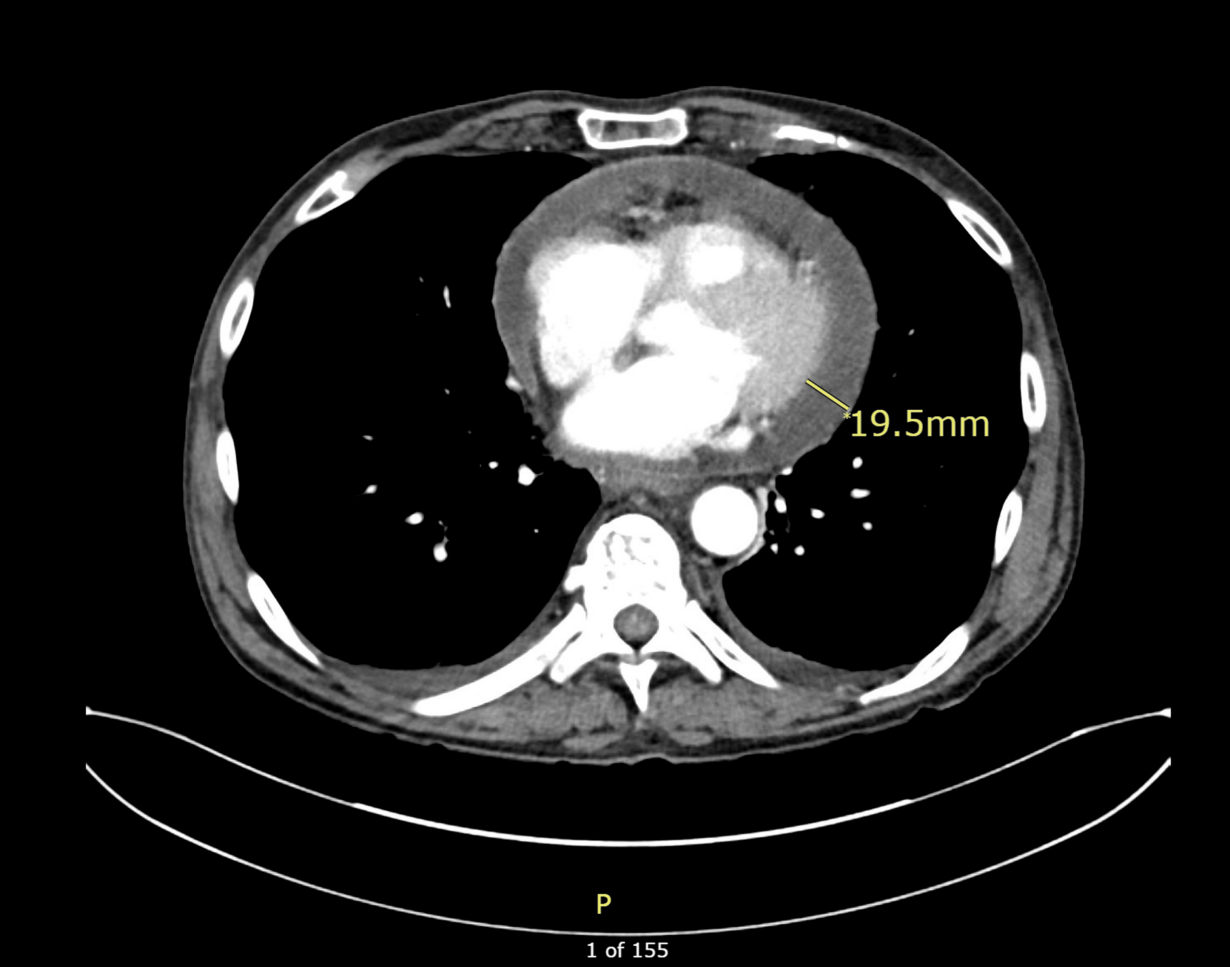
Contrast-enhanced axial CT scan demonstrating pericardial effusion The measurement marker indicates pericardial fluid thickness of approximately 19.5 mm.
